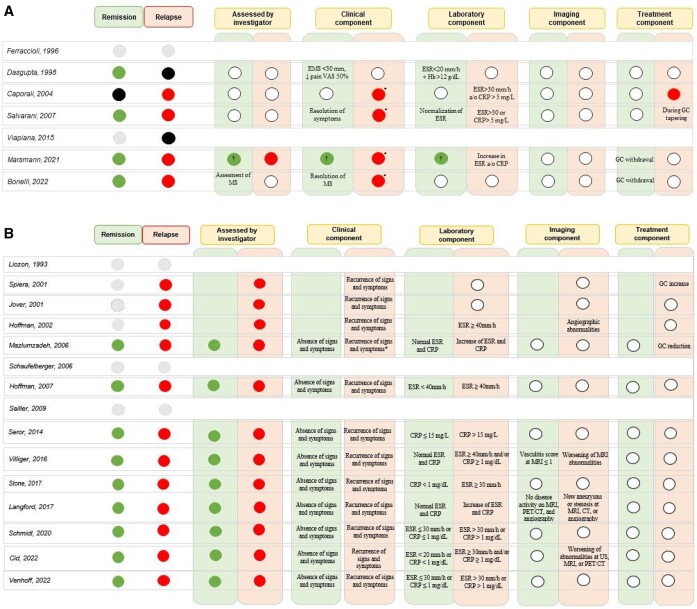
Components used in defining remission and relapse in (A) PMR RCTs# and (B) GCA RCTs#. Grey circle: Remission and/or relapse used as outcomes but not defined in the study methods; Green circle: remission (or specific components) defined in the study methods; Red circle: relapse (or specific components) defined in the study methods; White circle: component not part of the definition of remission/relapse. Black circle: remission/relapse not used as an outcome. CRP, C-reactive protein; CT, computerized tomography; EMS, early morning stiffness; ESR, erythrocyte sedimentation rate; GC, glucocorticoids; Hb, haemoglobin; MS, morning stiffness; MRI, magnetic resonance imaging; PET, positron emission tomography; US, ultrasound; VAS, visual analogue scale. *, signs and symptoms of active polymyalgia rheumatica; †, remission defined by PMR-AS (PMR Activity Score) <10; a/o, and/or. #Only RCTs considering remission and/or relapse as an outcome are listed. Overall, in (A) both remission and relapse were mainly defined as a combination of clinical and laboratory parameters. None of the studies defined sustained remission. In (B) relapse was defined as the return of signs and symptoms and/or an increase of ESR/CRP after reduction of prednisone dosage followed by an improvement of signs and symptoms when GC dosage was increased. Recurrence was defined as the reappearance of GCA signs and symptoms and/or increase of the inflammatory markers in a GCA patients not receiving GC therapy for at least 1 month. Overall, in GCA RCTs, both remission and relapse were mainly defined as a combination of clinical and laboratory parameters
